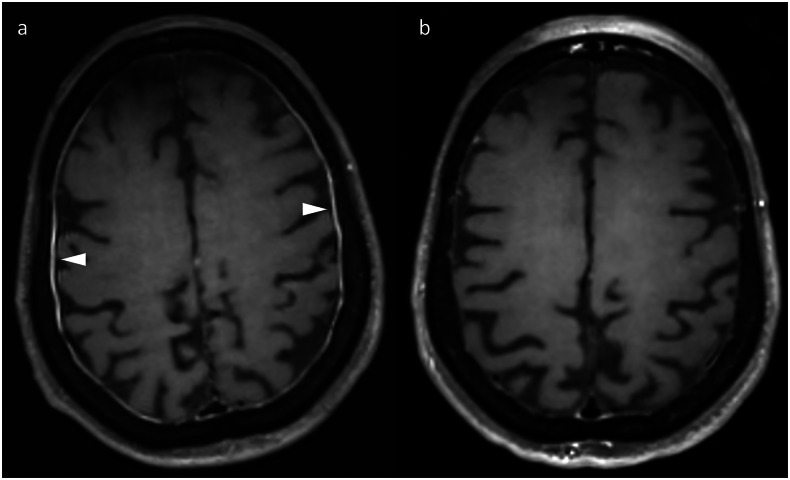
Serial axial post-contrast T1-weighted images in a patient who developed clinical features of irAE-meningoencephalitis while on maintenance nivolumab for metastatic melanoma. The images show abnormal pachymeningeal enhancement (a, arrowheads), which subsequently resolved after treatment (b)
